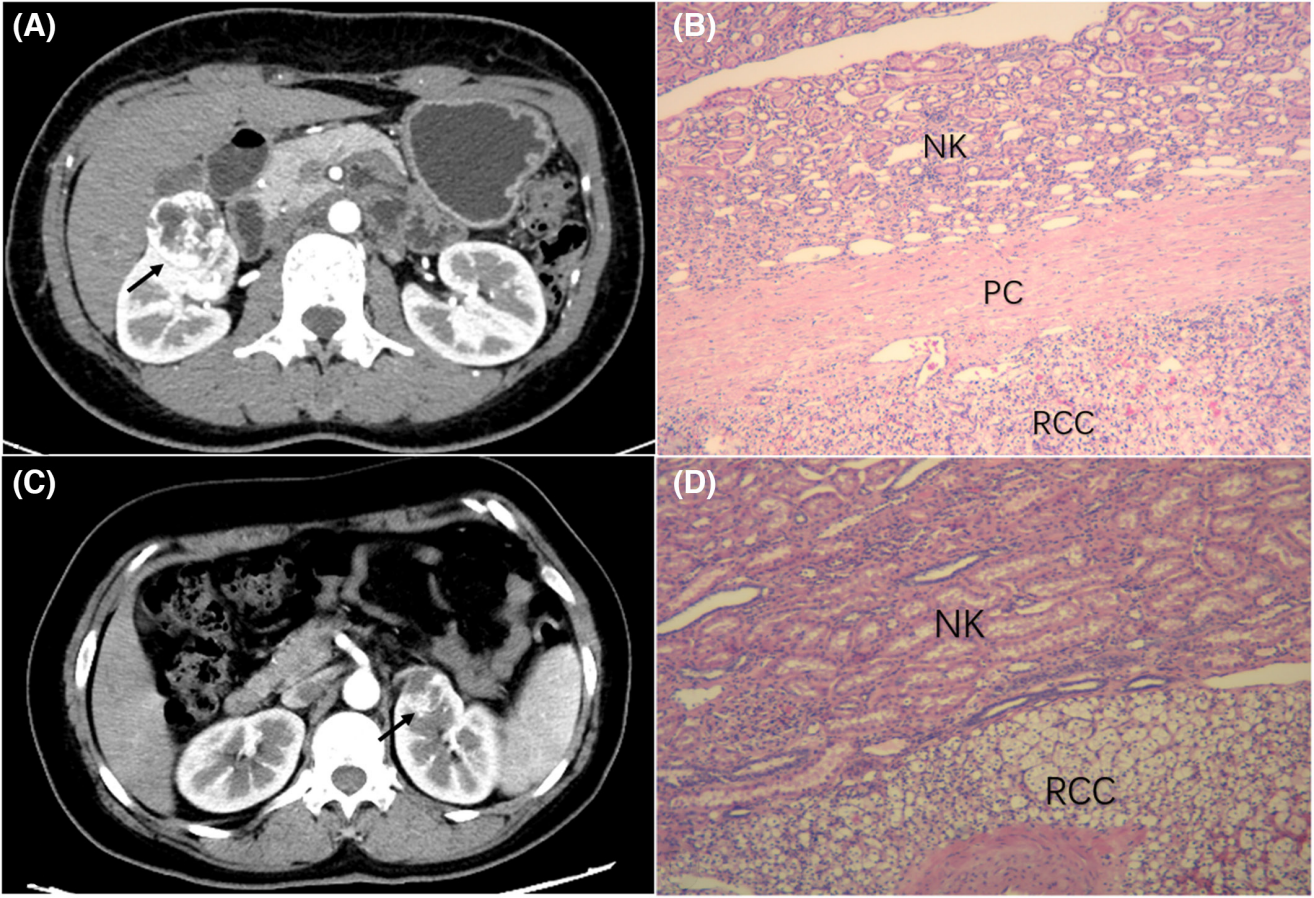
The typical computed tomography imaging feature and PC status in the clear cell renal cell carcinoma (A–D). Clear boundary (arrows) is observed at the tumor‐parenchyma interface on the corticomedullary phases image (A). Pseudocapsule is completely intact at the tumor‐parenchyma interface (B). Unclear boundary (arrows) is observed at the tumor‐parenchyma interface on the corticomedullary phases image (C). Pseudocapsule is absent at the tumor‐parenchyma interface (D). NK, normal kidney; PC, pseudocapsule; RCC, renal cell carcinoma
